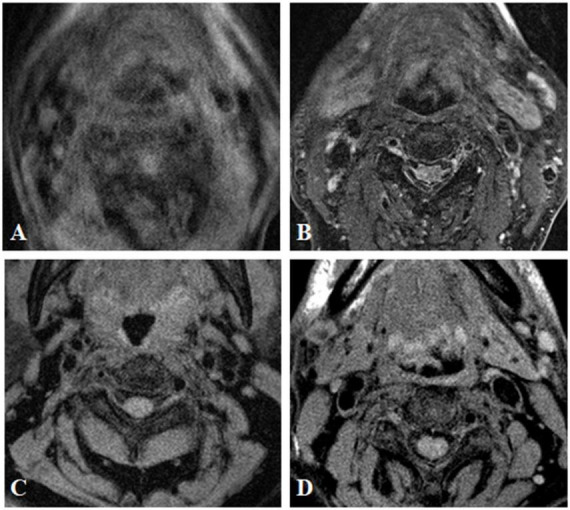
MR image quality evaluation of carotid plaque. The image quality of transverse axial T2WI showed carotid plaque was divided into grade 1 (A) grade 2 (B) grade 3 (C), and grade 4 (D).
